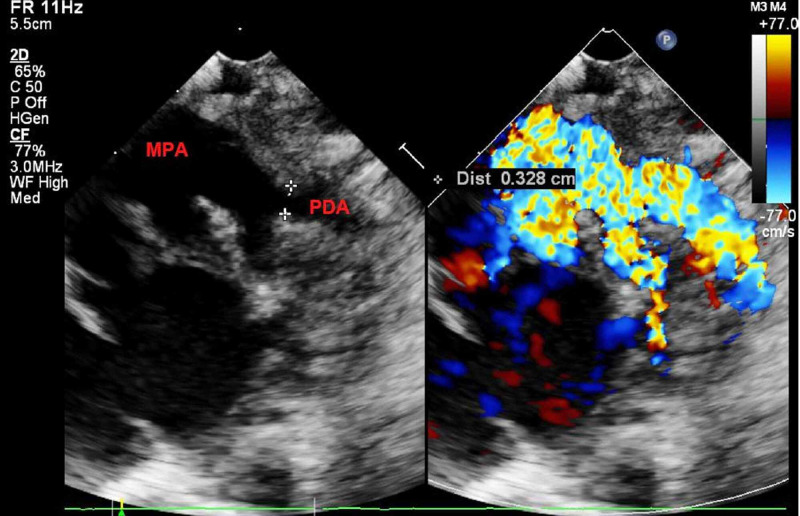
Two dimensional and color flow mapping of high parasternal “ductal” view showing large patent ductus arteriosus. MPA, main pulmonary artery; PDA, patent ductus arteriosus
